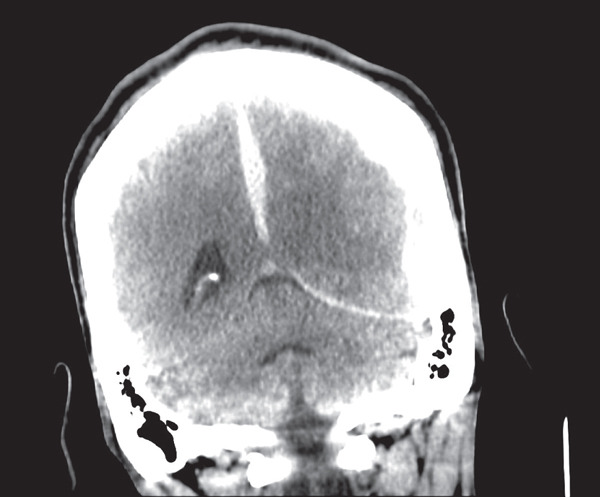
CT brain without contrast, horizontal view.
